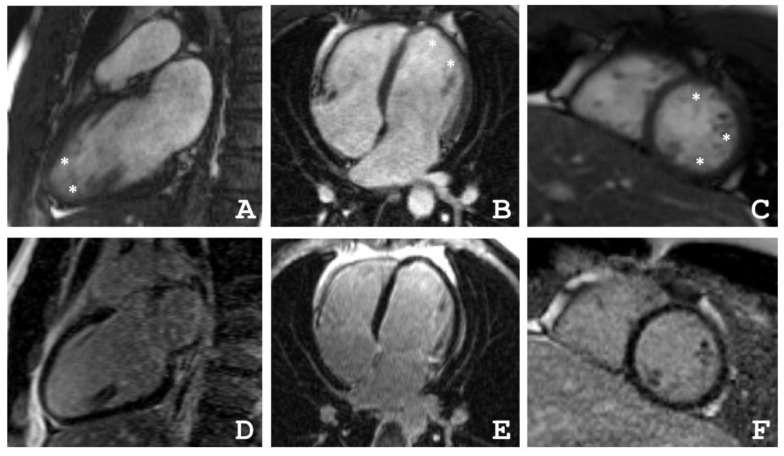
(A–C) Cardiac magnetic resonance imaging of the proband’s brother (III-2) in movie mode, SSFP sequence: (A) long axis 2-chamber projection, (B) long axis 4-chamber projection, (C) short axis at the level of apical segments, * indicates the layer of non-compacted myocardium, (D–F) delayed contrast. Inversion recovery sequence with suppression of the signal from the myocardium. There were no areas of contrast, which indicates the absence of areas of intra-myocardial fibrosis, scarring, and inflammatory myocardial damage.
